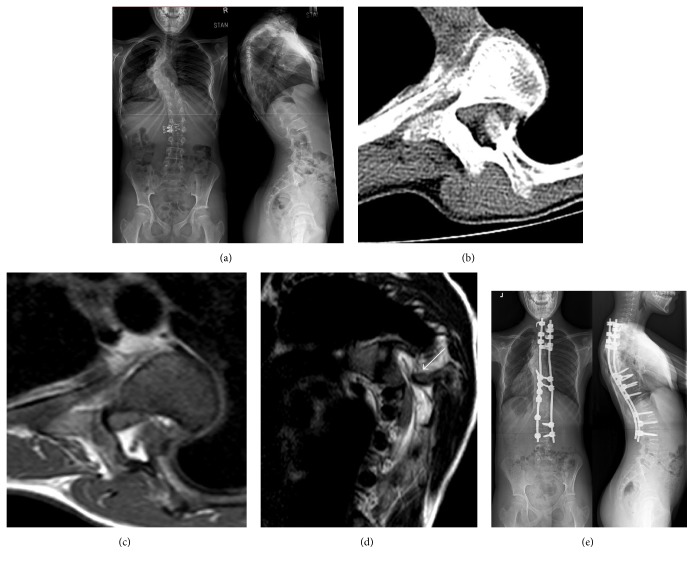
Preoperative whole spine AP and lateral X-ray (a). Preoperative CT (b) and axial T2 MRI (c) demonstrating the right 6th rib head intracanal dislocation and cord impingement. (d) Preoperative sagittal T2 MRI views demonstrating the rib head cord impingement and the flattening adjacent to the acute kyphosis (white arrow). Whole spine AP and lateral (e) X-rays 2 years postoperatively.
